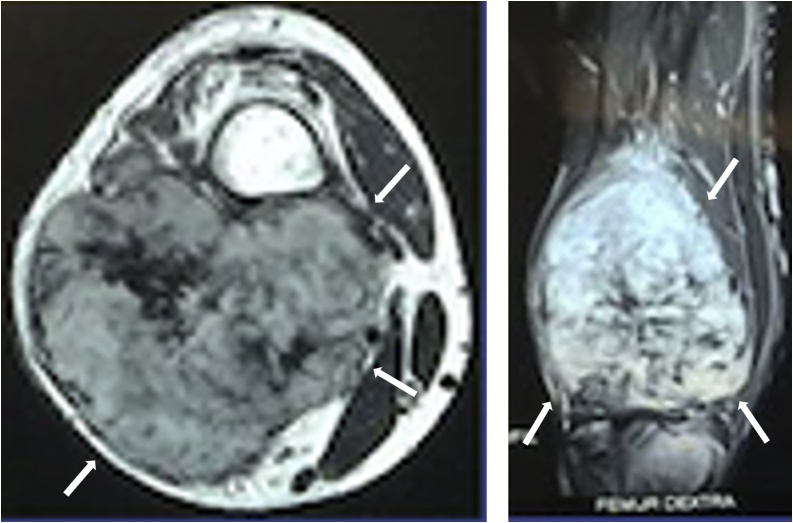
MRI revealed soft tissue mass sized 10 × 9 × 7 cm at posterior compartment of knee (white arrow).
